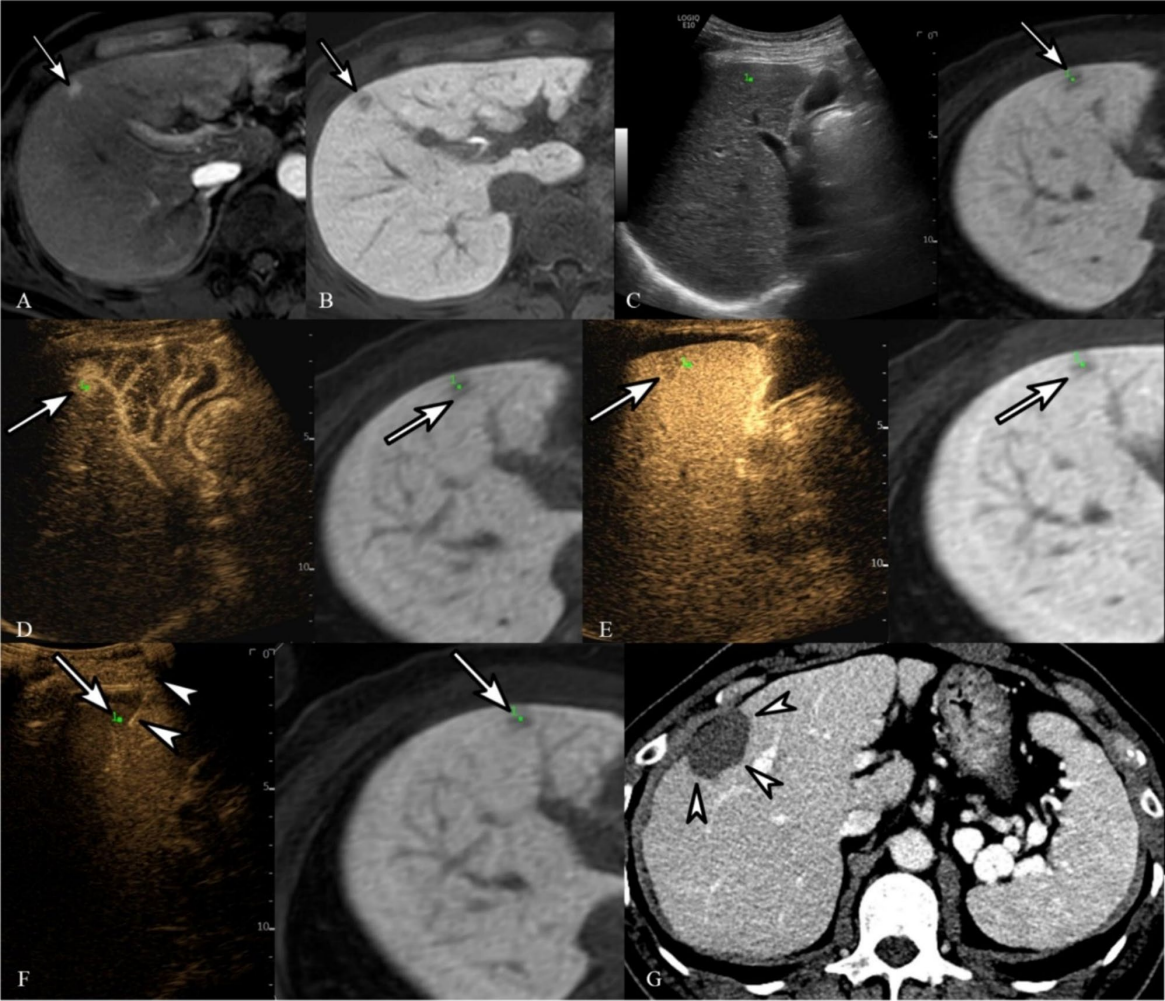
Images of using Sonazoid in radiofrequency ablation. Images of a 58-year-old man who had a 1.1-cm hepatocellular carcinoma (HCC) and hepatitis B virus-related liver cirrhosis and underwent CEUS-CT/MRI FI-guided radiofrequency ablation (RFA) with the use of Sonazoid as a contrast agent. (A) Arterial-phase MRI demonstrates a small hypervascular HCC (arrow) at the border of liver segments 5 and 8. (B) In hepatobiliary-phase (20-min delayed phase) MRI, the tumor at segment 5/8 border of the liver showed an apparent defect (arrow). (C) Following fusion imaging, it was not possible to identify the HCC on B-mode US imaging at the corresponding site on fused MRI (arrow). Therefore, the index tumor was assigned a conspicuity score of 1, as it was unidentifiable on fusion imaging. (D) Arterial-phase imaging obtained post-Sonazoid injection demonstrated an identifiable small enhanced lesion (arrow) at the corresponding location on fused MRI. (E) Kupffer-phase imaging identified the HCC (arrow) at the corresponding location on fused MRI. Therefore, the index tumor was assigned a conspicuity score of 4 on CEUS-added fusion imaging. (F) Radiofrequency electrodes (arrowheads) were positioned in the index tumor (arrow) with CEUS-CT/MRI FI guidance. (G) LTP was not identified at the site of ablation on portal-phase CT obtained 17 months post-RFA (arrowheads)
